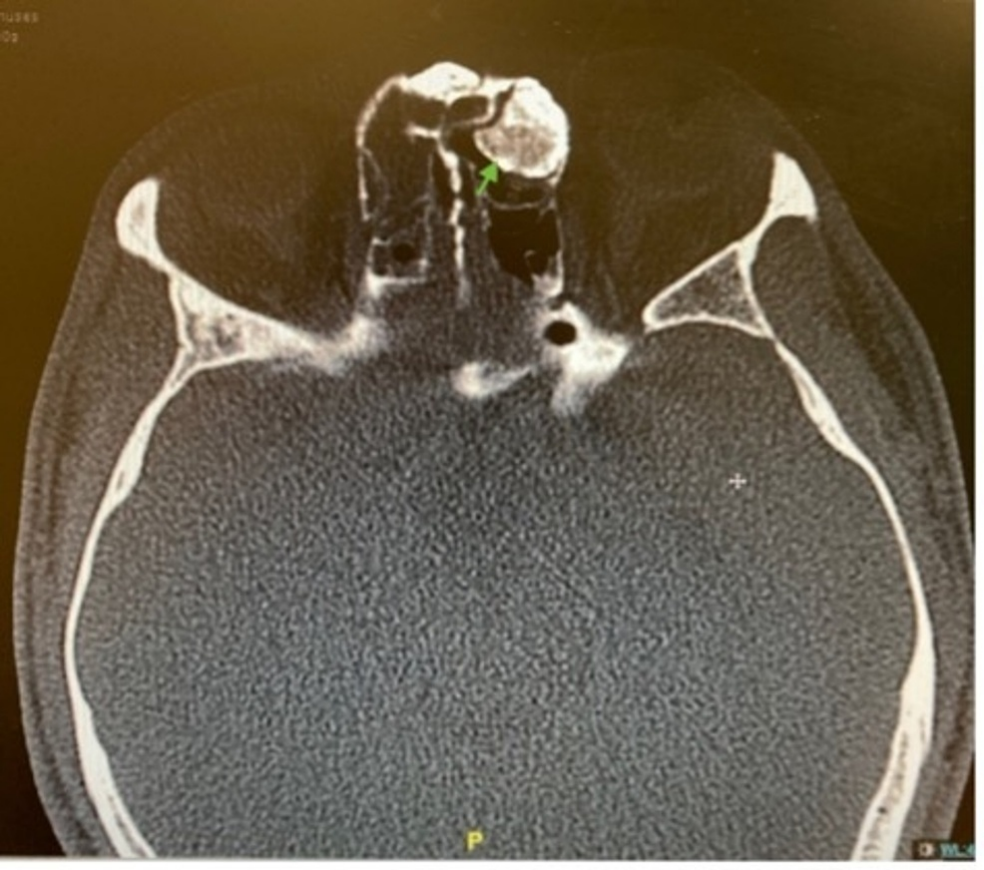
Axial view of the patient’s CT PNS The osteoma is indicated by the green arrow. CT: computed tomography; PNS: paranasal sinus
